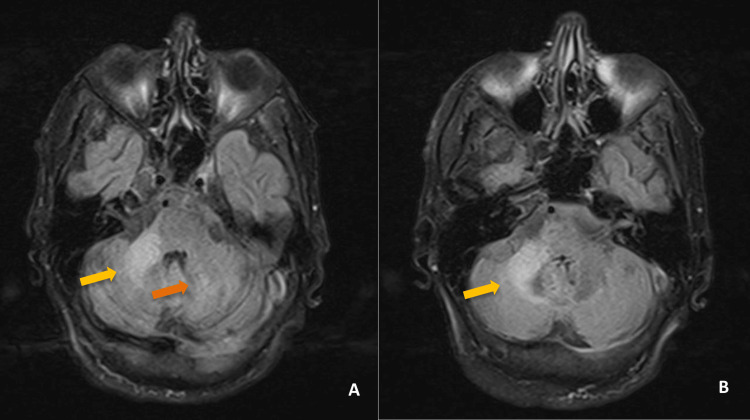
Second brain MRI 1 month after the previous one, transverse T2/FLAIR sequence (A and B) with increased size of the peduncular lesion (yellow arrows) and appearance of a new lesion on the contralateral cerebellar hemisphere with the same characteristics (orange arrow). FLAIR: fluid attenuated inversion recovery
